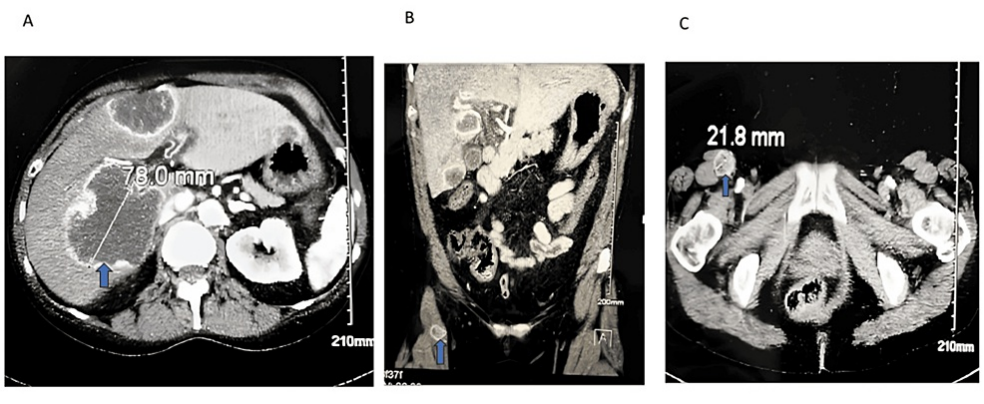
A CT scan of the abdomen and pelvis with IV contrast showed a large hepatic metastatic lesion (3A and 3B) and a right sartorial muscle lesion measuring 21.8mm (3C).
